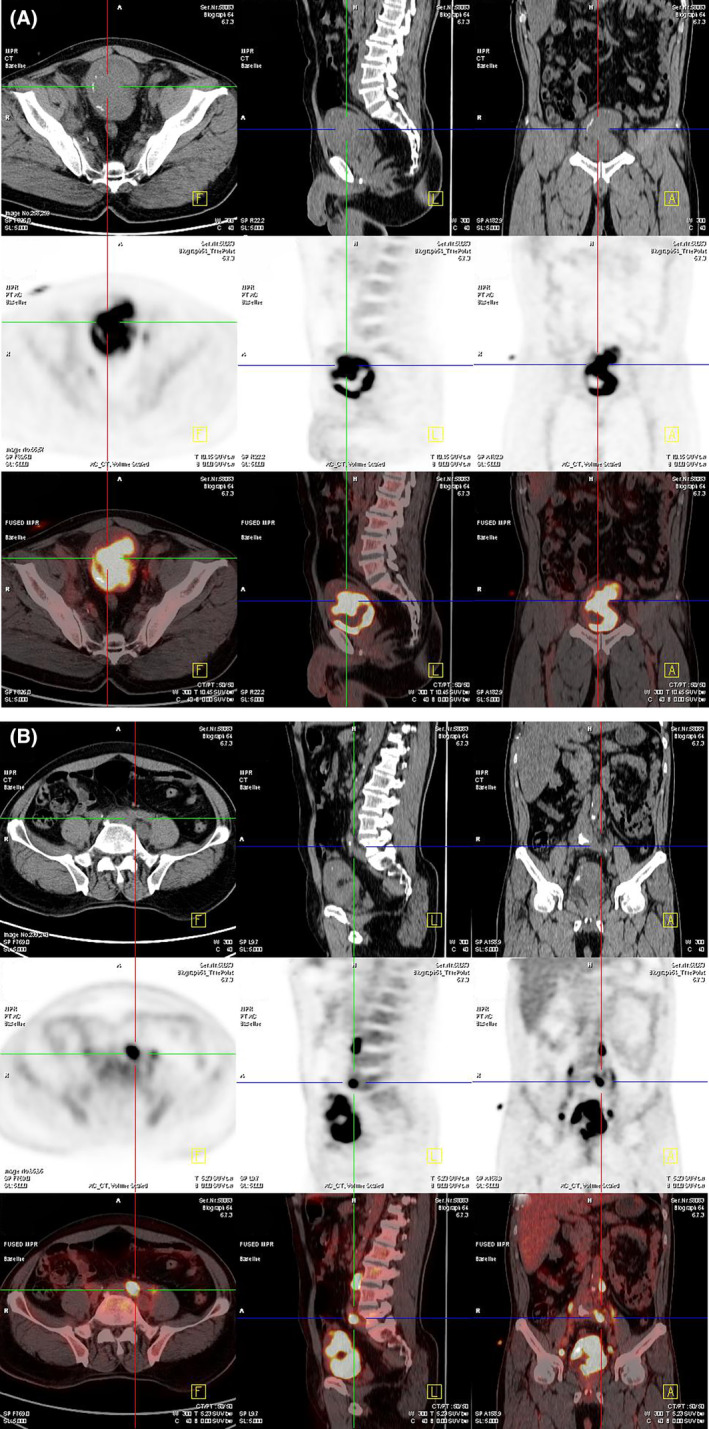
Positron emission tomography‐computed tomography of sarcomatoid carcinoma of the urinary bladder. (A) Positron emission tomography‐computed tomography demonstrated that the irregular mass exhibited heterogeneous density and striped calcification. (B) Multiple enlarged lymph nodes were observed in the lower abdomen, adjacent to the abdominal aorta and bilateral iliac vessels.
